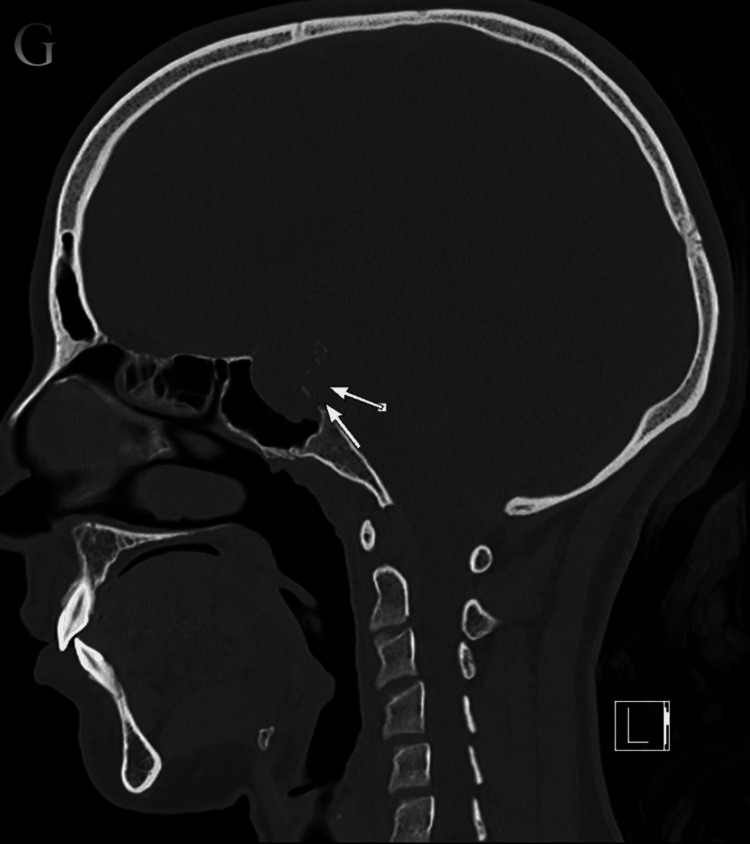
In the brain CT on sagittal plane bone window (G), the lesion was causing destruction (long arrows) of the pituitary fossa, dorsum sellae, and tuberculum CT: computed tomography
